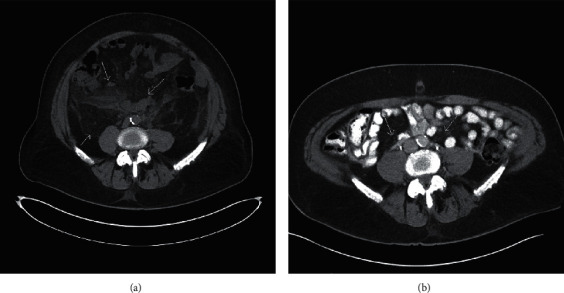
CT scan abdomen. (a) Initial and (b) follow-up scan 1 year later after discontinuation of etanercept, without systemic steroids.
